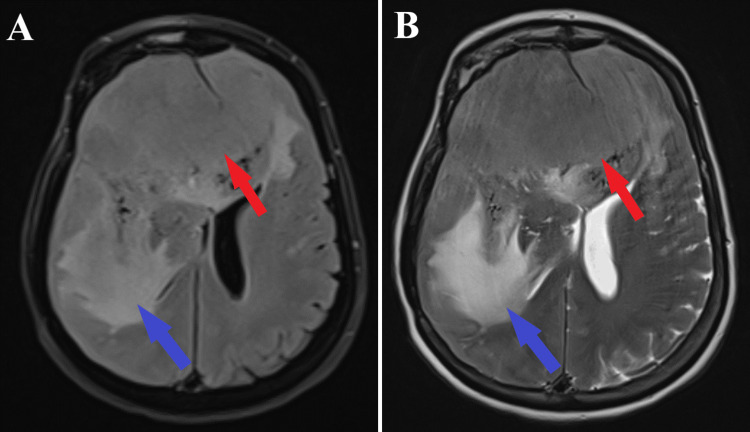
Preoperative MRI T2 and T2 FLAIR sequence, axial section. A: MRI T2 sequence showing the tumoral mass (red arrow) and significant perilesional edema (blue arrow).
